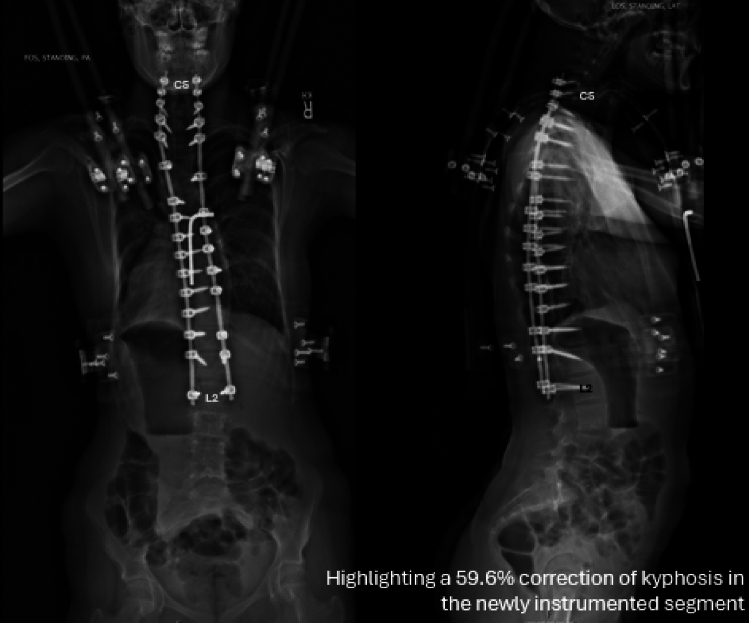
Case 1: Postoperative PA and lateral X-ray of a 12-year-old female with DiGeorge syndrome and syndromic scoliosis
